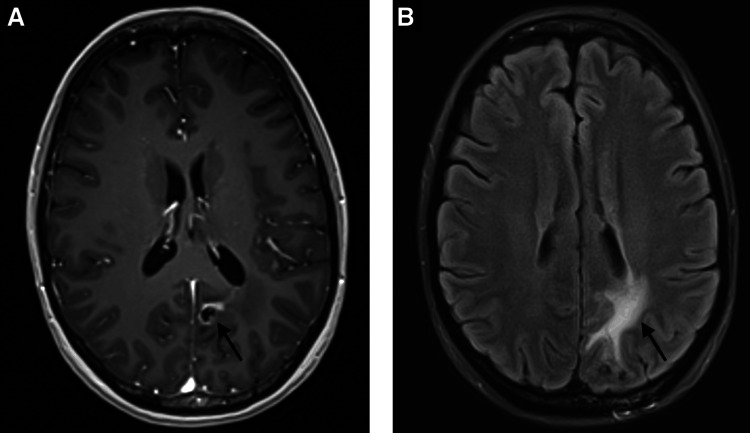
One-year post-treatment T1 with contrast (A) and T2-FLAIR (B) axial MRI sequences. Post-treatment imaging demonstrating minimal residual enhancement and no evidence of progressive disease (A; arrow indicates post-radiation changes). Surrounding T2 FLAIR hyperintensity is reduced and consistent with decreased vasogenic edema and treatment effect secondary to RT (B; arrow indicates reduced vasogenic edema).
